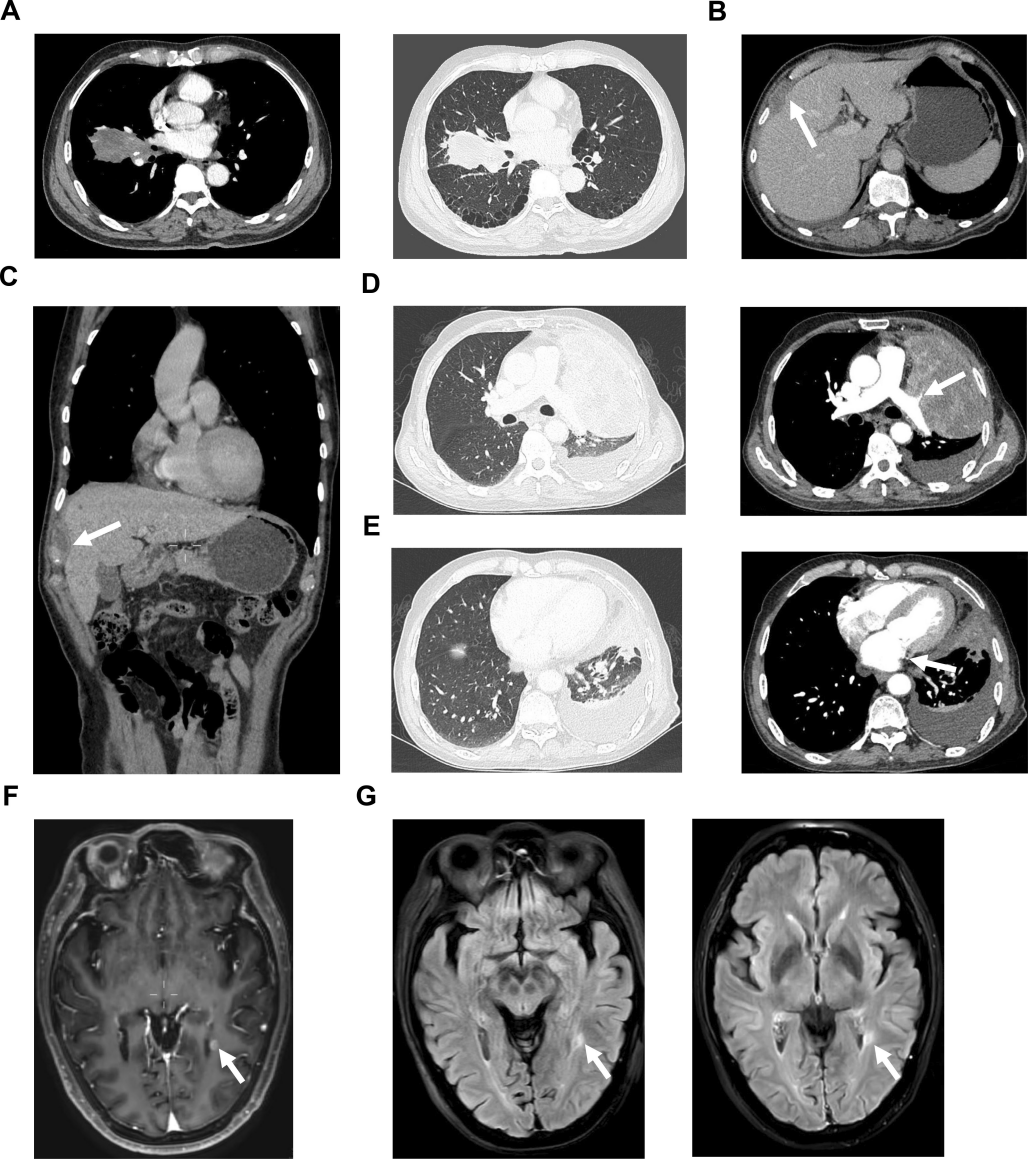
Images revealing diaphragmatic metastasis of Case 2 and brain metastasis of Case 3. (A) Chest CT at diagnosis of Case 2. (B) Low density observed in the diaphragmatic muscle at the right margin of the liver in Case 2. (C) Coronal view displaying a low‐density shadow in the diaphragm of Case 2 detected by chest CT. (D) Pulmonary occupation with invasion of pulmonary vessels indicated by chest CT at diagnosis of Case 3. (E) Involvement of the left atrium shown on chest CT of Case 3. (F–G) T1‐weighted signal mass (F) and T2‐weighted signal mass based on FLAIR images (G) indicated nodular enhancement in the brain parenchyma around the trigone of the left lateral ventricle in Case 3, suggesting the presence of metastatic tumor. Arrows indicate masses. CT, computed tomography; FLAIR, fluid‐attenuated inversion recovery; MRI, magnetic resonance imaging.
